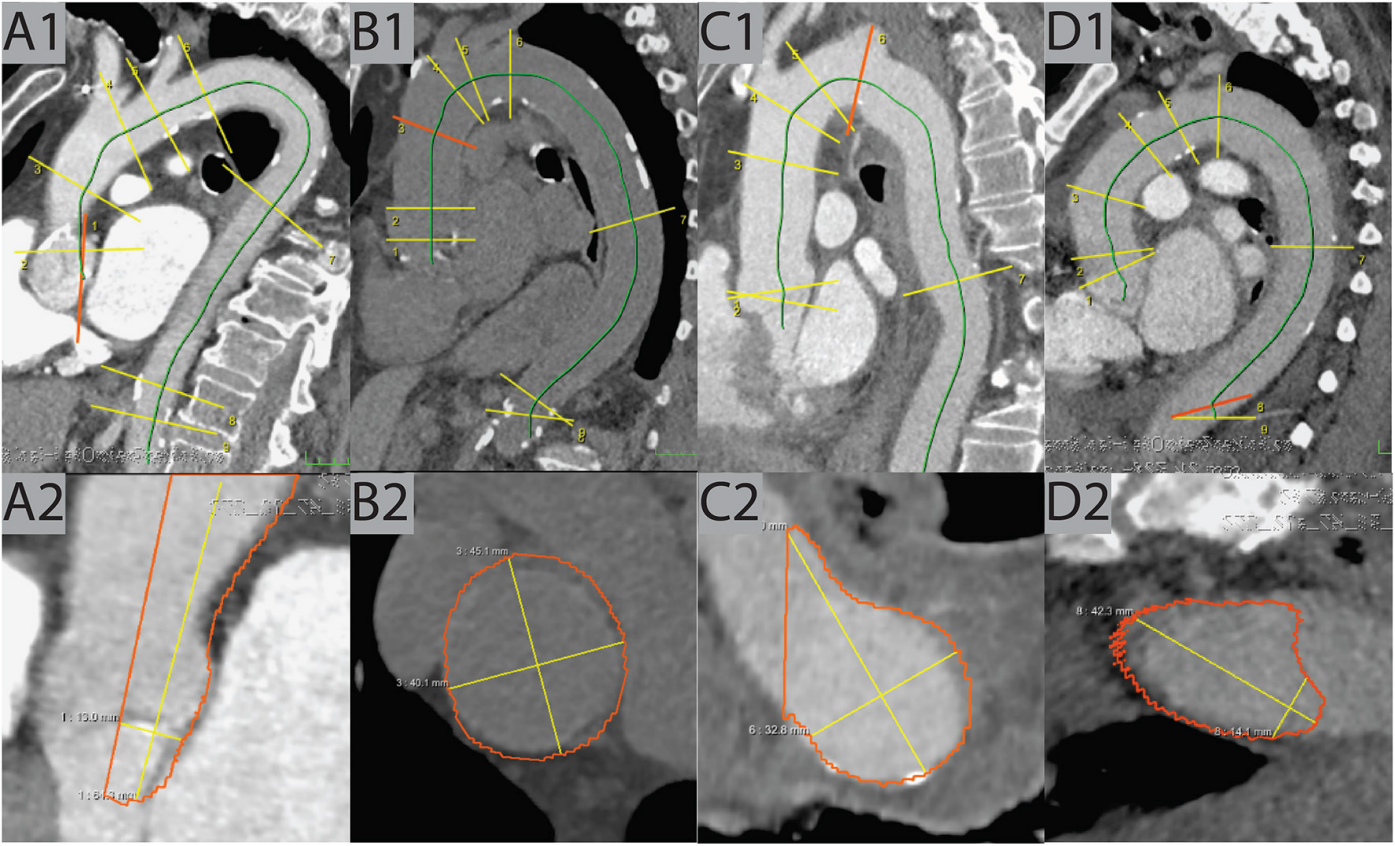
Examples of false positive cases. (A) Due to an error in centerline placement, the AS plane was tilted and falsely contoured, resulting in a false-high measurement in this pulmonary-arterial scan of a 93-year-old female patient. (B) In this non-CE scan of an 82-year-old female patient, the contouring at the AA location was too wide, resulting in a false-high measurement. (C) The CT in pulmonary-arterial phase of 75-year-old male patient showed an aberrant right subclavian arteria. This caused an error contouring the location of the DA, resulting in a false-high measurement. (D) The last two locations (DDA, ABA) in the pulmonary-arterial phase CT of an 85-year-old female patient were tilted caused by an erroneous centerline placement, resulting in a false-high measurement at DDA (42 mm). AA, ascending aorta; ABA, abdominal aorta; AS, aortic sinus; CE, contrast enhanced; DA, distal arch; DDA, distal descending aorta.
